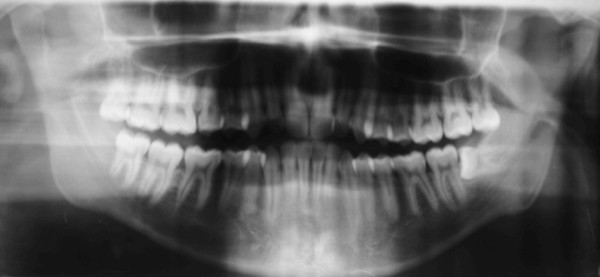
Panoramic radiograph revealing enhanced density of the cortical layer over the left condyle 8 months after commencement of treatment.
